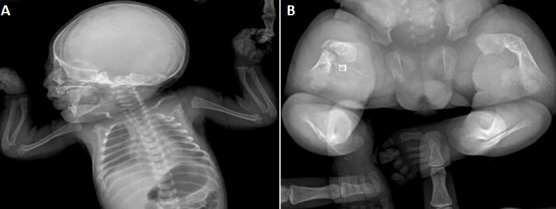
Radiographie de squelette après la naissance; (A) défaut d'ossification du crâne, membres supérieures très courts, côtes déformées porteuses de cals, (B) membres inférieures courts et incurvés
